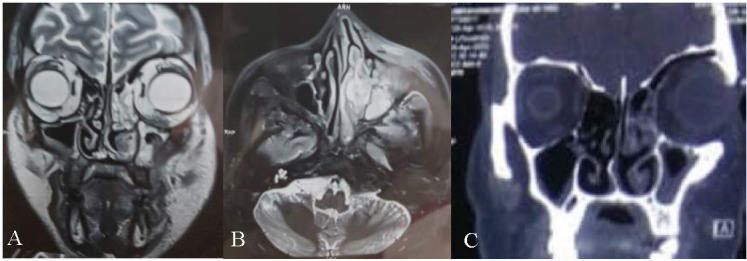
A. T2 weighted coronal section of MRI showing left frontal, ethmoidal and maxillary sinusitis with periantral extension into the premaxillary soft tissues, involving the left nasal cavity and the middle and inferior turbinates. B. axial MRI image showing involvement of the left infratemporal and pterygoid involvement along with ethmoidal and maxillary sinusitis. C. Coronal section of CT PNS showing moderate mucosal thickening in the inferior left frontal sinus, left maxillary and left ethmoidal sinus
